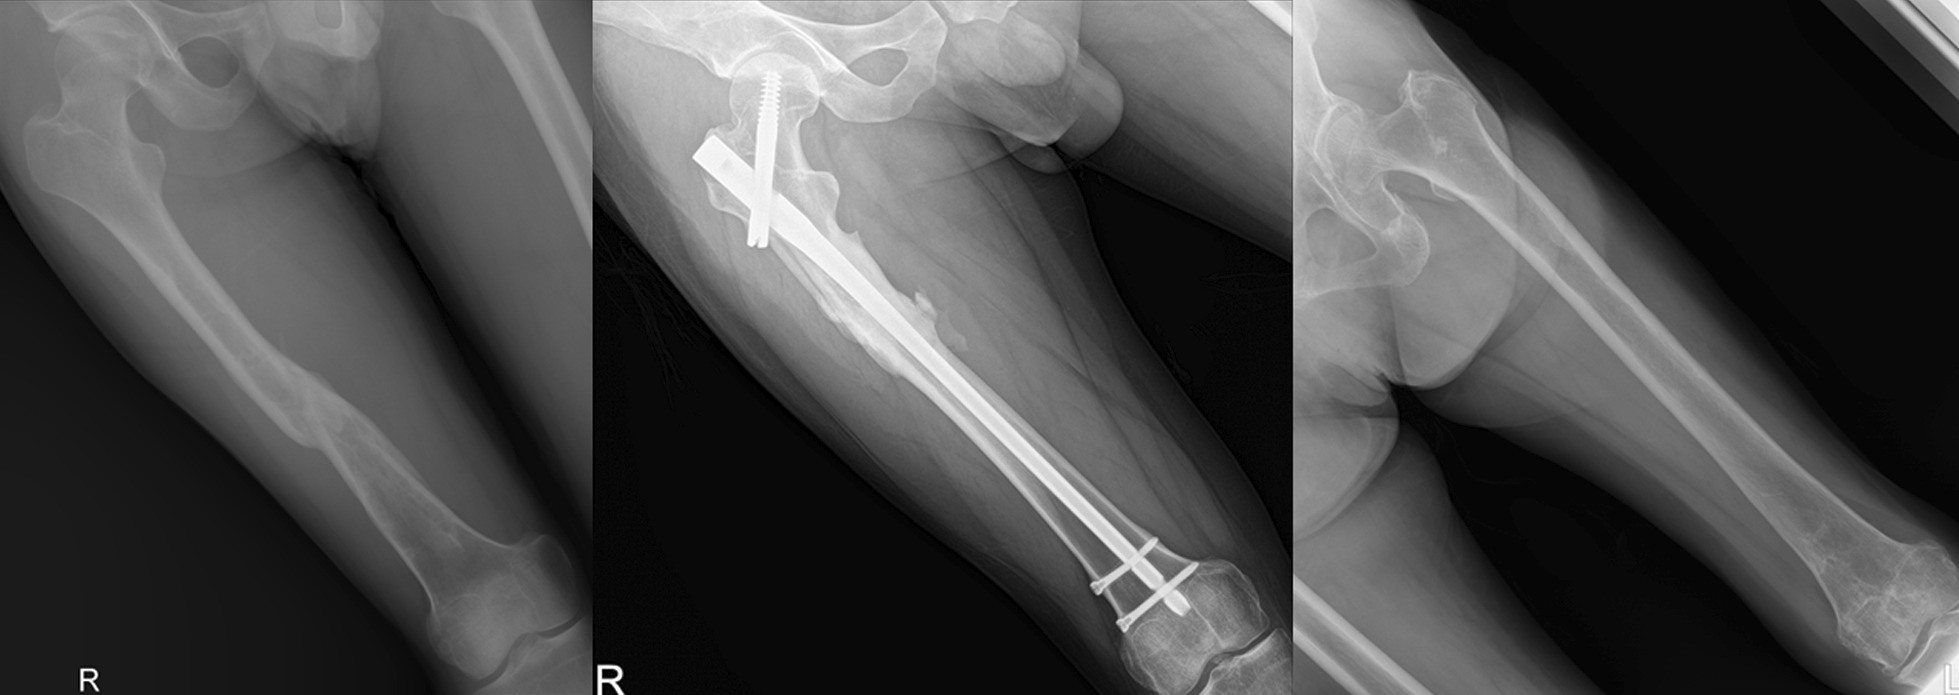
Cases requiring the evaluation of rotational deformity after femoral fractures. Although each fracture was successfully treated and healed, it was difficult to assess rotational deformity without CT imaging
